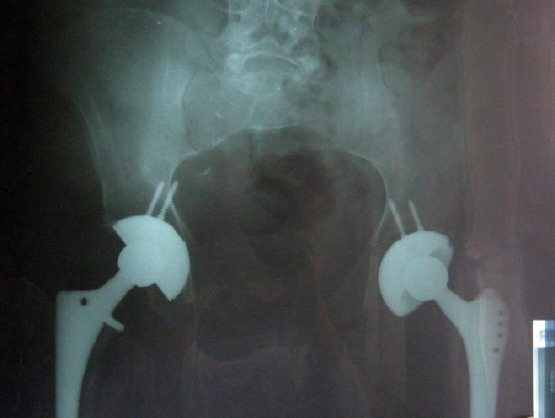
X-ray of pelvis with both hips after both total hip artroplasty*.
